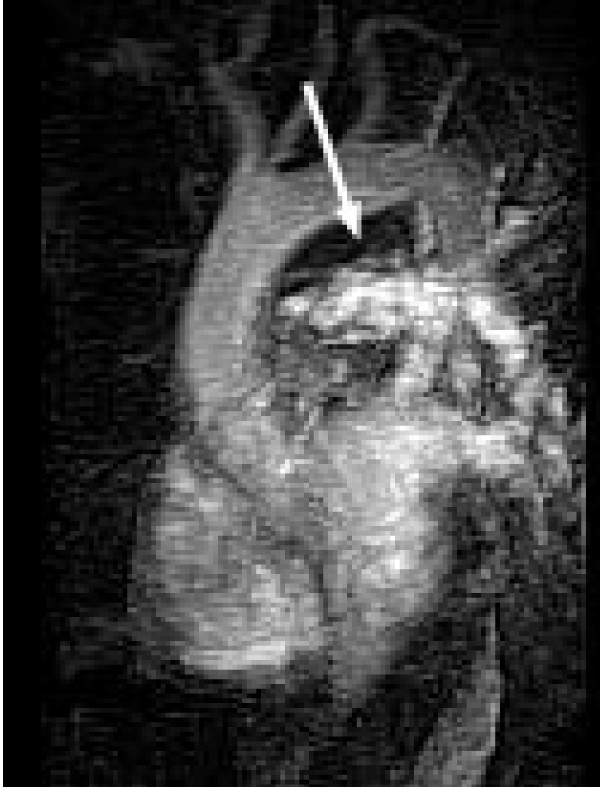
Cardiac MRI showing the sarcoma arising from right ventricle outflow tract to both the right and left pulmonary artery.
